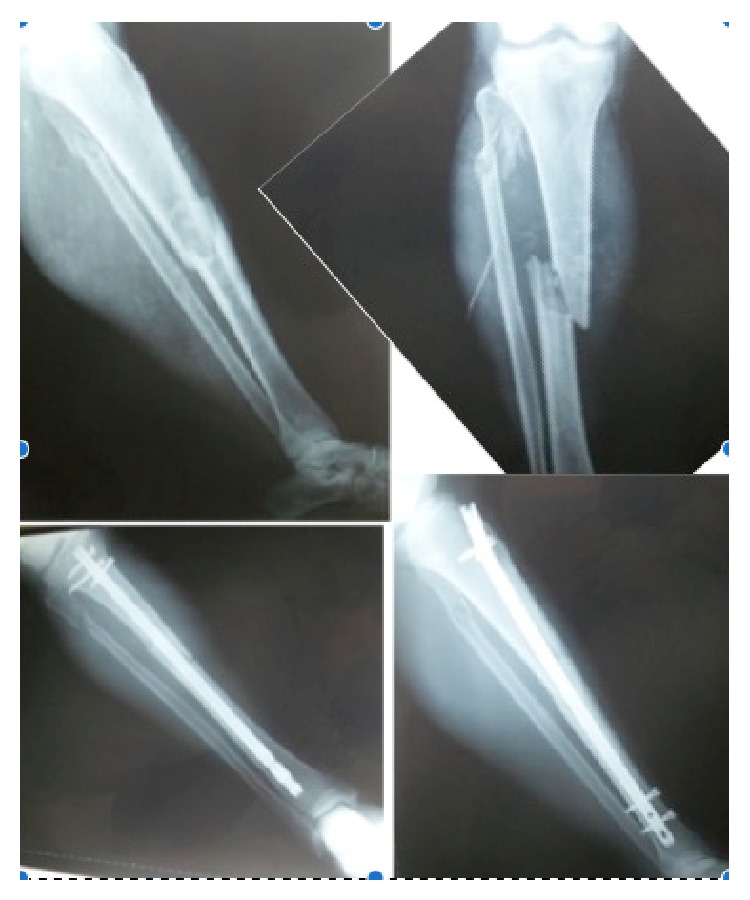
Anteroposterior and lateral X-ray images showing a middle one-third tibial shaft fracture with an associated fibular fracture and a healed tibial fracture treated with intramedullary nailing.
